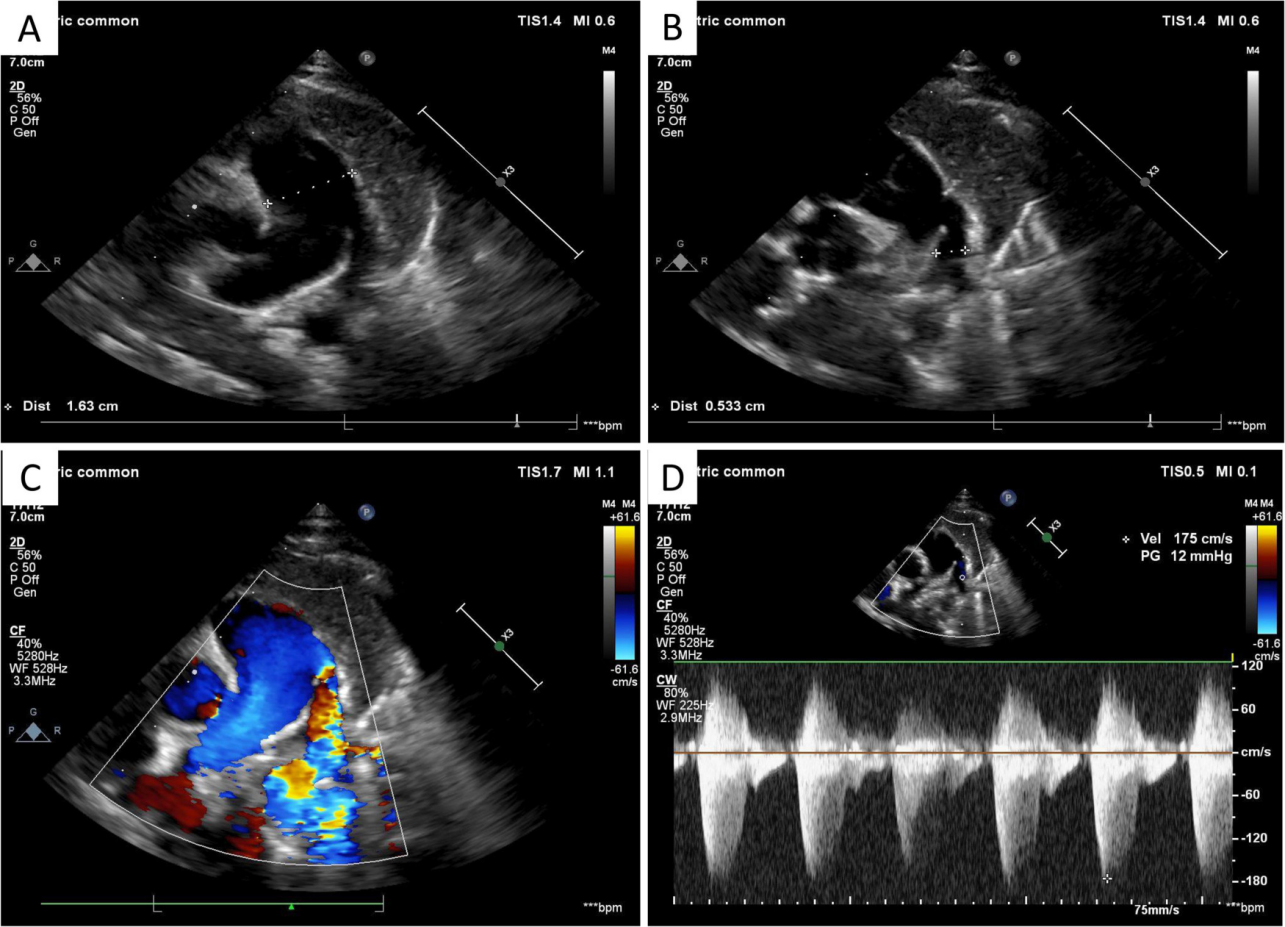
Postoperative bedside TTE. (A) Parasternal short-axis view showing the MPA, which measured 1.63 cm in diameter, indicating a reduction from the preoperative size. (B) 2D echocardiography view of the reimplanted LPA (arrow) originating from the side wall of the MPA, with a patent proximal diameter of 0.533 cm. (C) CDFI demonstrating patent blood flow through the LPA anastomosis with appropriate color filling. (D) Continuous-wave Doppler interrogation showing a peak flow velocity of 1.75 m/s (pressure gradient 12 mmHg) across the LPA anastomosis, consistent with a widely patent surgical connection in the early postoperative period.
